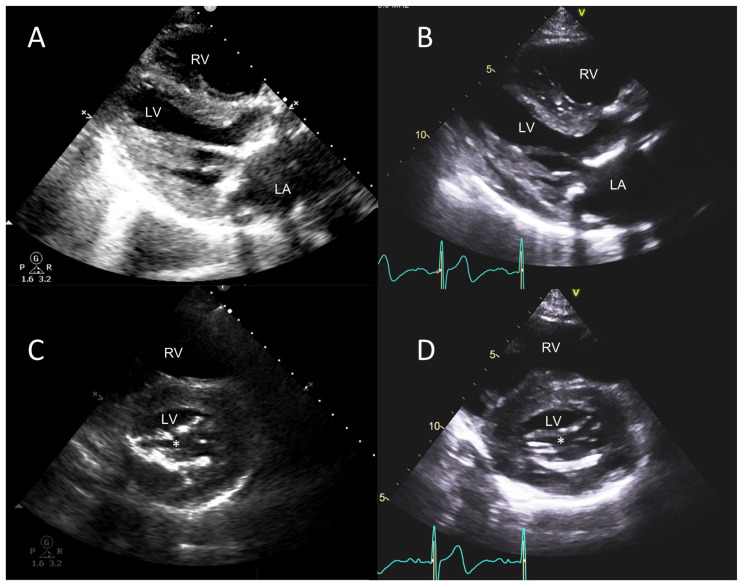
POCUS in stenotic mitral VHD. A 85-year-old male with history of biprosthetic aortic valve implant 8 years ago presented with dyspnea on exertion. POCUS images (left-side panels) are compared to the sTTE images (right-side panels). Parasternal long axis views (A,B) showing marked calcification of mitral leaflets extending from aortic sub annular region, and of the mitral annulus as well as subvalvular apparatus. Parasternal short axis views (C,D) in diastole showing marked calcification and restriction of leaflet mobility and reduction in mitral orifice area (white asterisks) suggestive of significant calcific MS. Severe mitral annular calcification is also shown. LA, left atrium; LV, left ventricle; RV, right ventricle.
